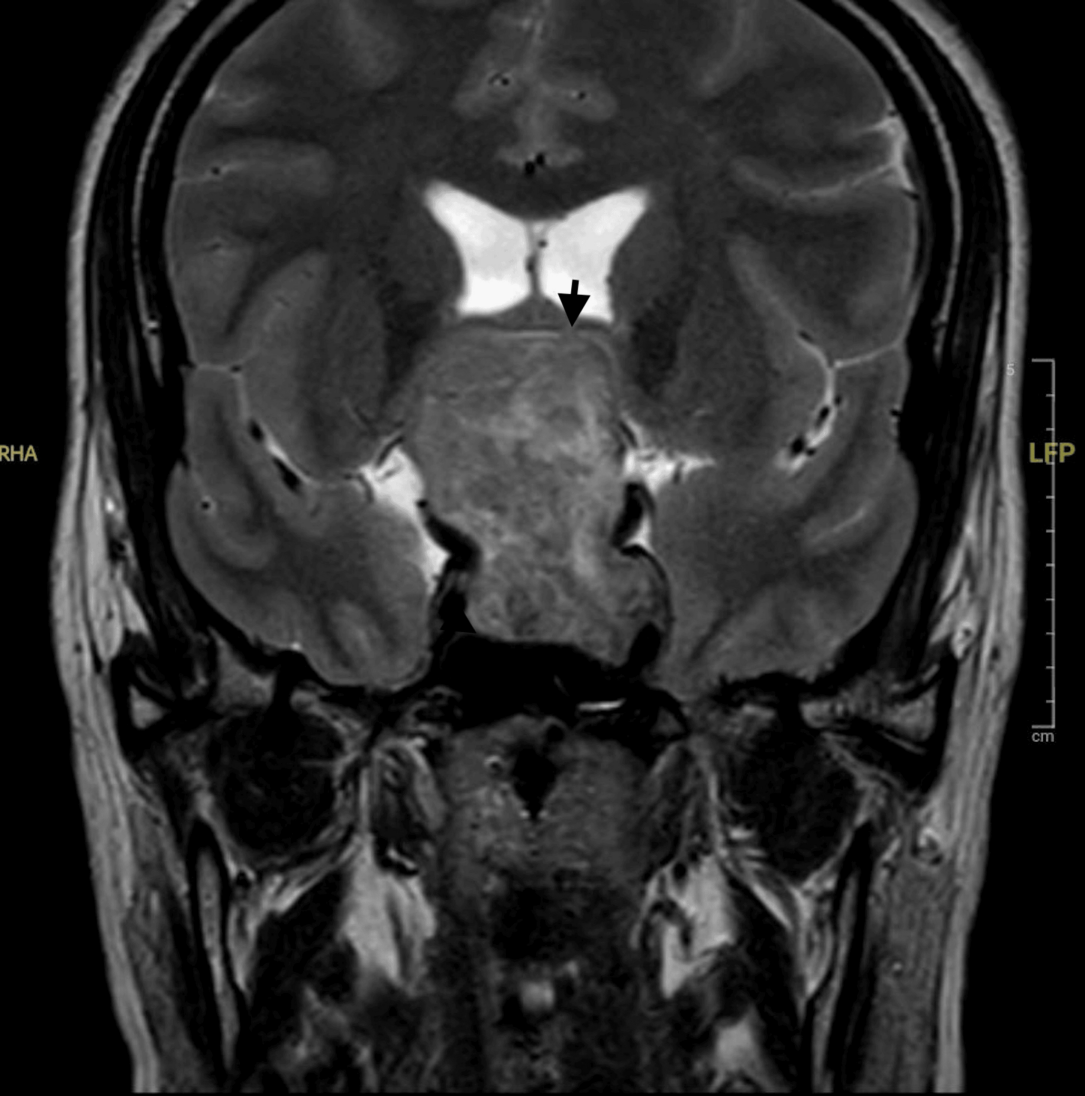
T2-weighted coronal section of the brain showing a large solid (black arrow), heterogenous sellar, and suprasellar mass lesion mixed hypo and intense signals. The pituitary gland is not seen separately
